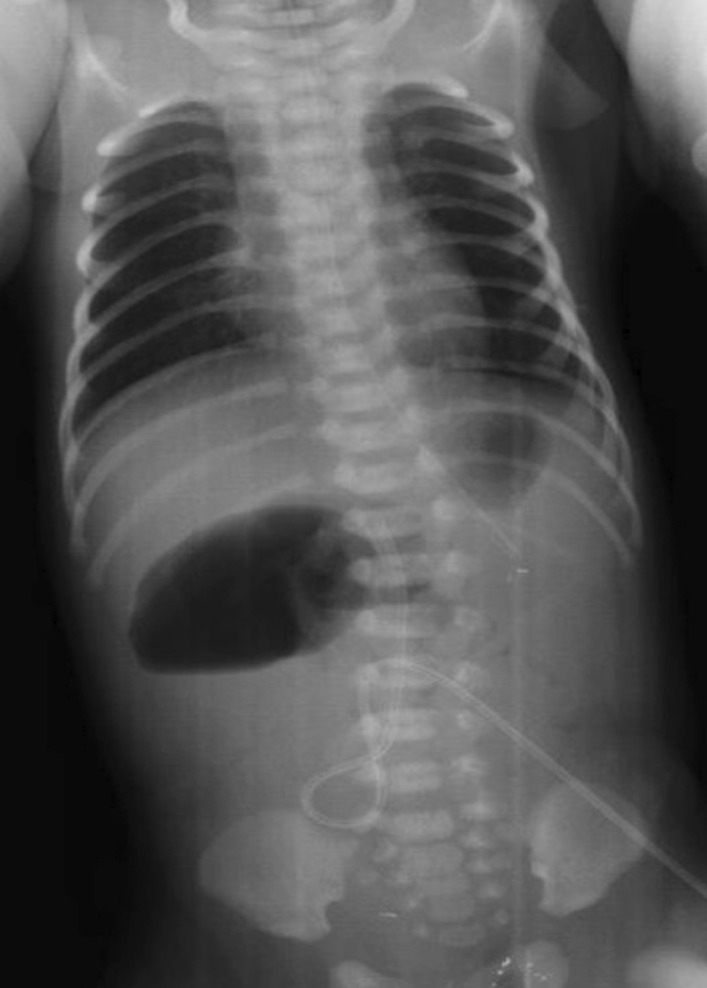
Air–fluid levels suggesting proximal obstruction on X-ray
